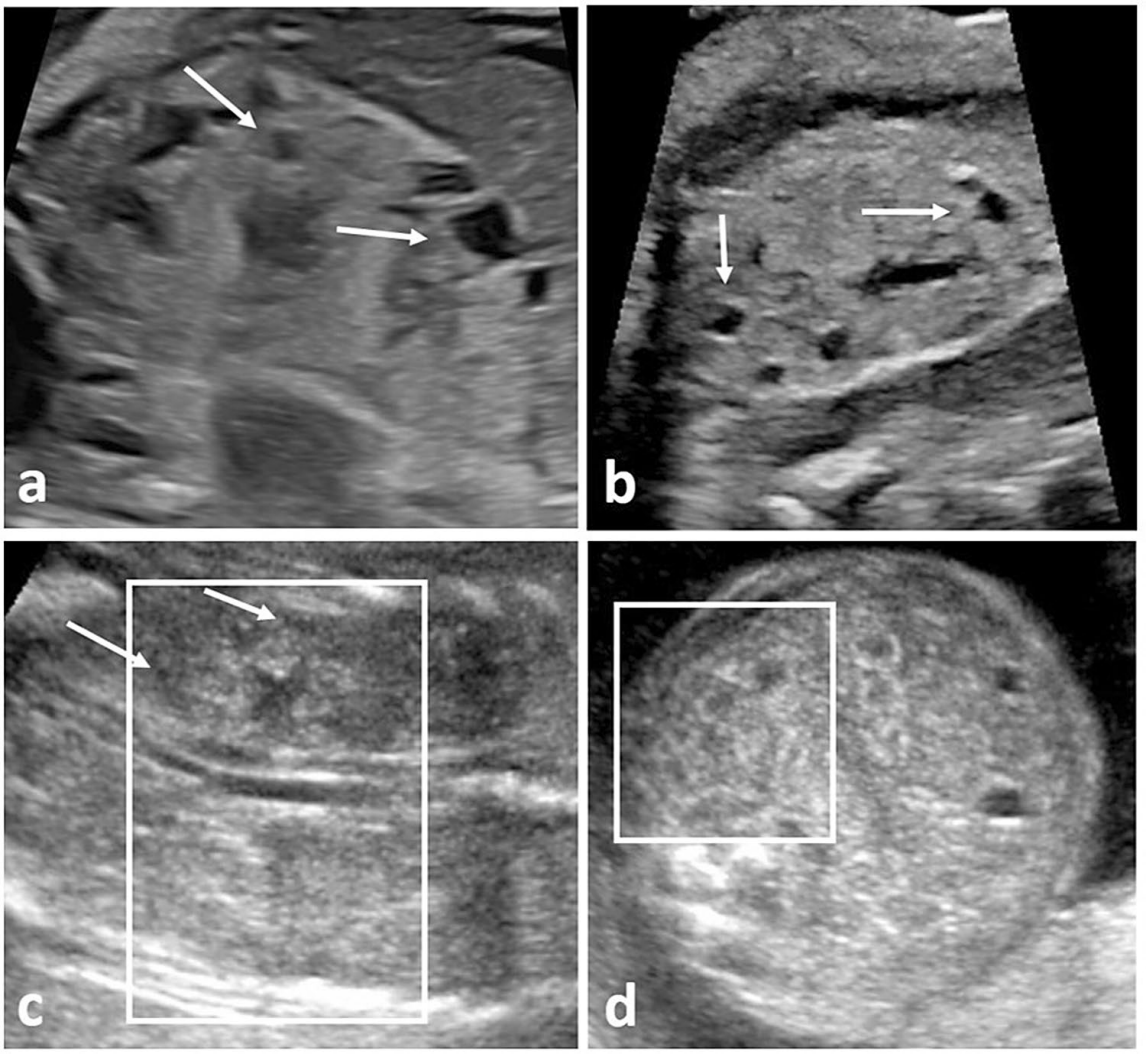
Prenatal ultrasound in fetuses with Mainzer–Saldino syndrome and Beckwith–Wiedemann syndrome (*BWS*). a+b Show a fetus with Mainzer–Saldino syndrome at 30+3 weeks’ (a) and 34+3 weeks’ (b): kidneys are enlarged, hyperechogenic, with absent *CMD* and small cortical cysts (white arrows); c+d show a fetus with BWS at 21+6 weeks’, presenting with absent *CMD* and small cysts (white arrows) located predominantly at the corticomedullary junction (coronary view and axial view – white box)
